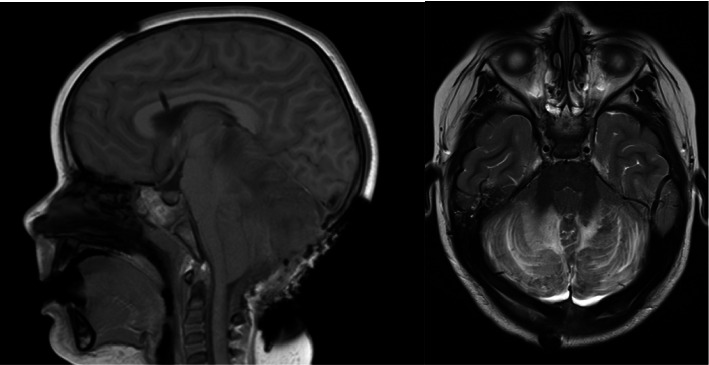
MRI (left T1 flair and right T2 TSE) status post-EVD insertion and suboccipital craniectomy. There remains severe posterior fossa mass effect owing to cytotoxic edema of the cerebellar hemispheres as described above. There are evolving supratentorial cytotoxic changes as described above, with new involvement of the globi pallidi.
